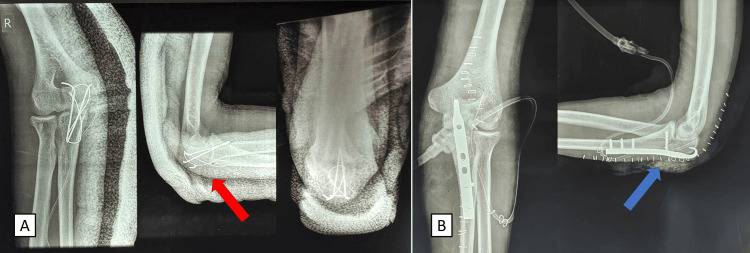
Immediate postoperative radiographs of the left and right elbows (A) Anteroposterior and lateral radiographs of the right side, with the tension band wiring done for the Mayo type I right olecranon fracture visible in the lateral view (red arrow); (B) anteroposterior and lateral radiographs of the left side, with an anatomical plate used to fix the Mayo type II left olecranon fracture visible in the lateral view (blue arrow).
